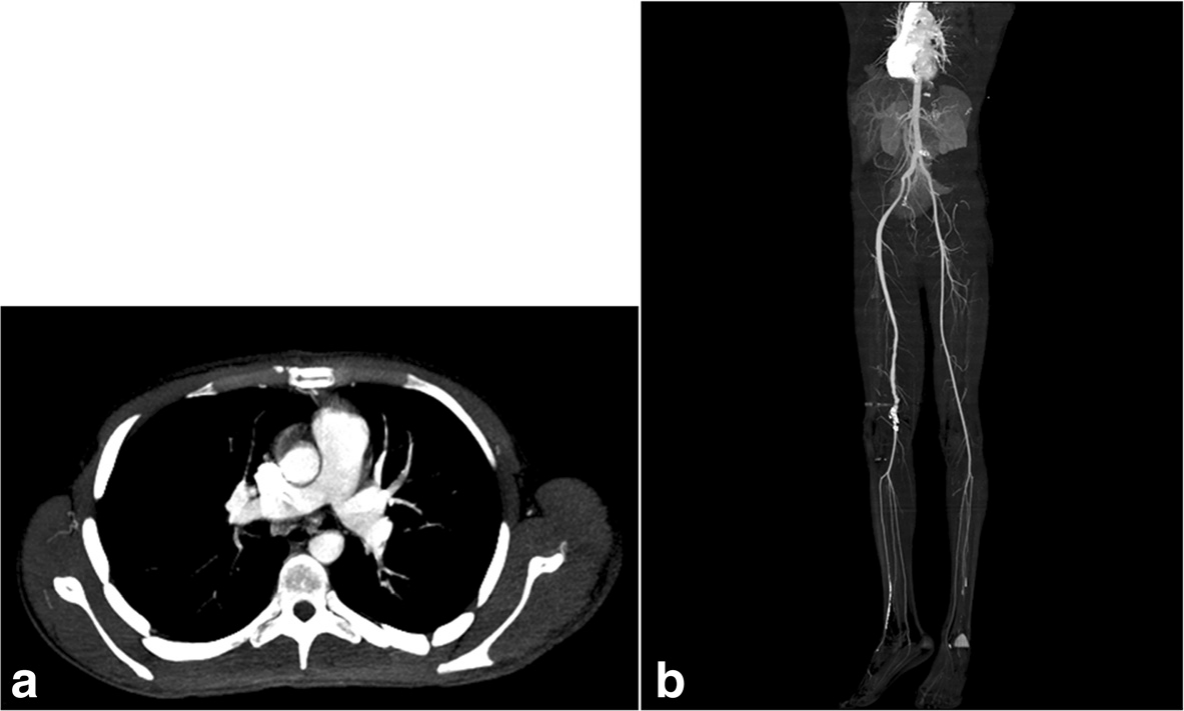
Postoperative CT angiography (a) showing a clear pulmonary arteries (b) patent peripheral arteries.
